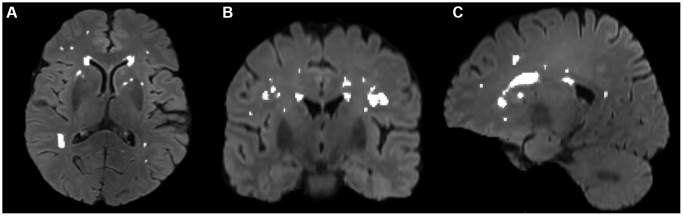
Segmentation of WMH volumes in the transverse (A), coronal (B), and sagittal (C) positions on FLAIR images. The white color represents the automatically segmented WMH range. WMH, white matter hyperintensities.
